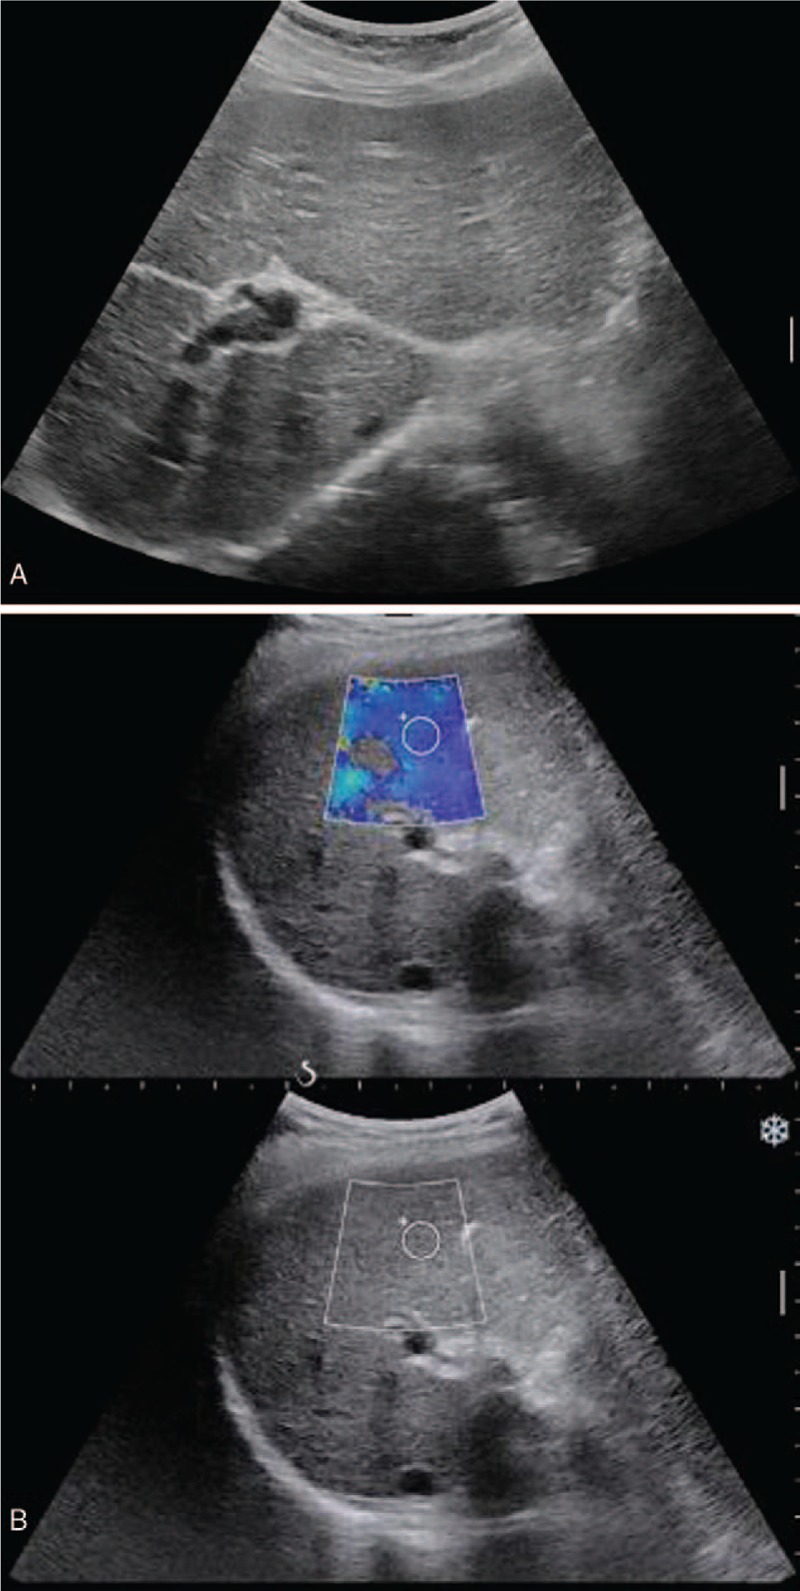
Ultrasound image of a 45-year-old woman with abnormal liver function. (A) Grayscale sonogram demonstrated coarse echotexture of the liver parenchyma and shrunken right lobe. (B) Cirrhosis was suspected with an elevated liver stiffness of 11 KPa measured by shear wave elastography.
